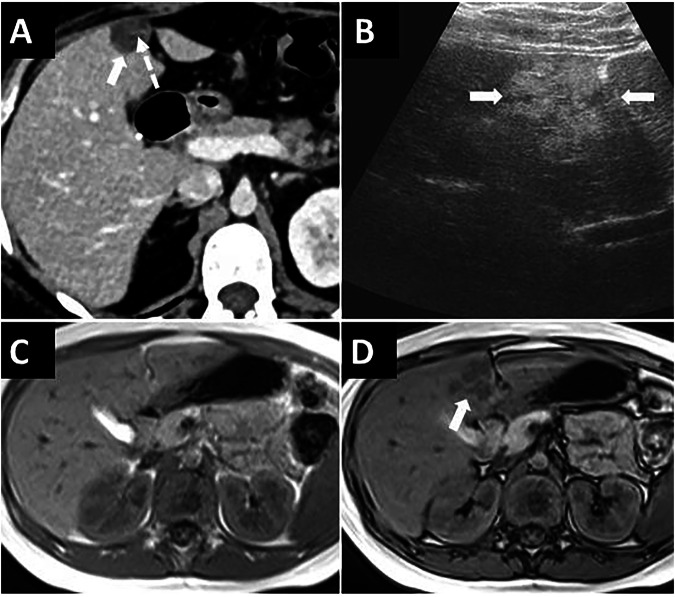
Focal fat near the FL. A 27-year-old male patient with a history of sleeve gastrectomy. Axial plane contrast-enhanced CT image shows focal fat (arrow) near the FL. Note the vessel traversing the lesion (dashed arrow). B–D 32-year-old female patient with known breast cancer. B Ultrasound image shows a hyperechoic lobulated lesion close to the FL (arrows). In-phase (C) and out-of-phase T1-weighted (D) MRI images confirm the lesion as a focal fat by the presence of a focal signal drop (arrow in D) on the out-of-phase image
